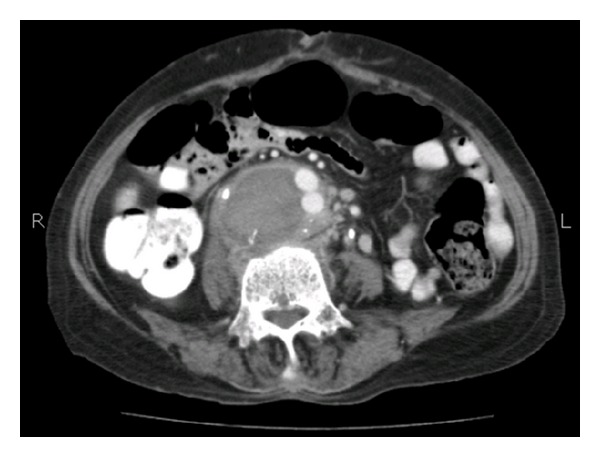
Enhanced CT obtained 15 days after embolization shows that the pseudoaneurysm is thrombosed.
